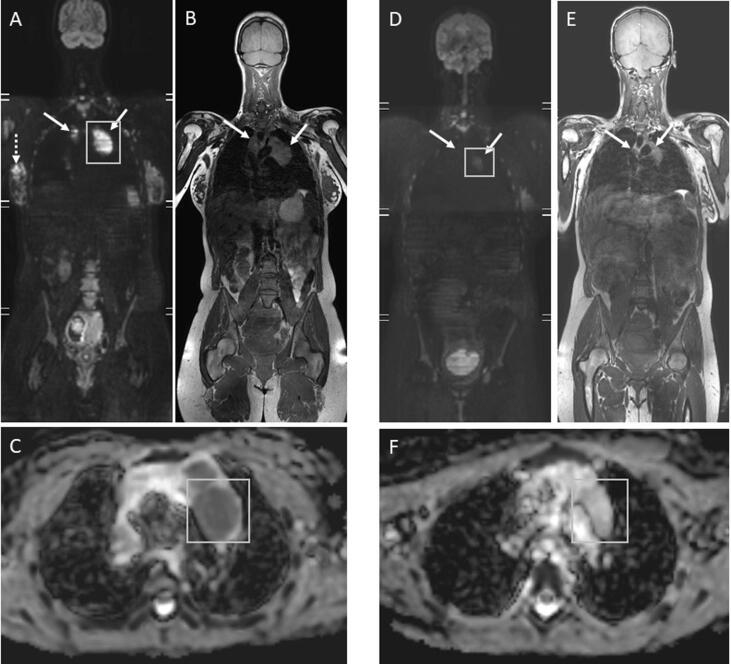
Whole body diffusion-weighted magnetic resonance (WB-DWI/MRI) in pregnant patient with aberrant noninvasive prenatal test (NIPT): (A) b1000 DWI shows enlarged and bright mediastinal lymphadenopathies (arrows) with anatomical correlate at (B) T1-weighted image indicative of lymphoma and a bright nodular lesion (dashed arrows) in de the right breast suspicious of breast cancer. (C) Corresponding apparent diffusion coefficient (ADC) map of the mediastinal lymphadenopathy (green box) shows value of 0.00089 mm^2^/sec confirming malignancy. Interim WB-DWI/MRI after 2 cycles of chemotherapy and right mastectomy shows (D) signal substantial loss at b1000 DWI and partial volume regression at (E) T1-weighted image of the mediastinal lymphadenopathies (arrows) corresponding to (F) a substantially increased ADC of 0.00191 mm^2^/sec indicating substantial necrosis induction and thus excellent response of the lymphoma.
